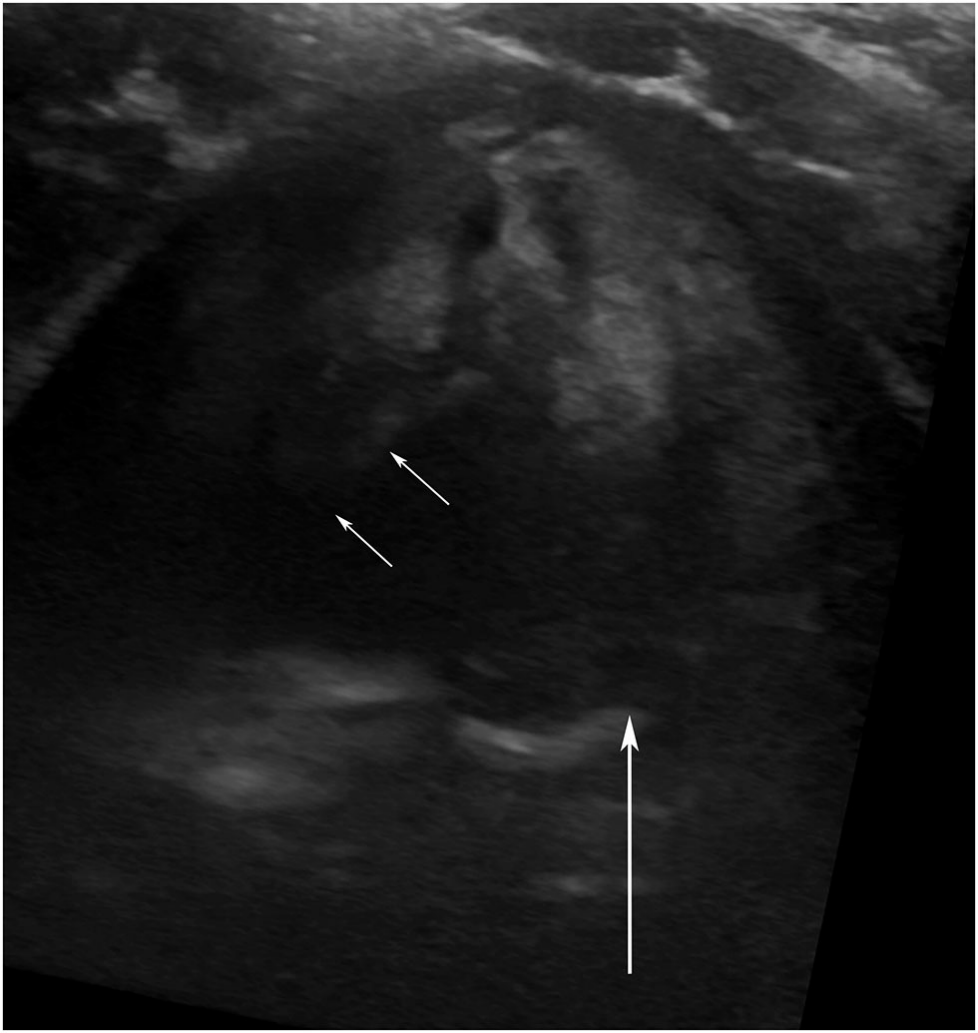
Vocal cord ultrasound. Transverse image of the larynx in a 3-year-old male 2 days after division of a vascular ring. The right vocal fold (small arrows) is abducted. The edge of the left vocal fold is hard to visualize (because it runs parallel to the ultrasound beam), but its adducted position is confirmed by the medial position of the left arytenoid cartilage (large arrow).
